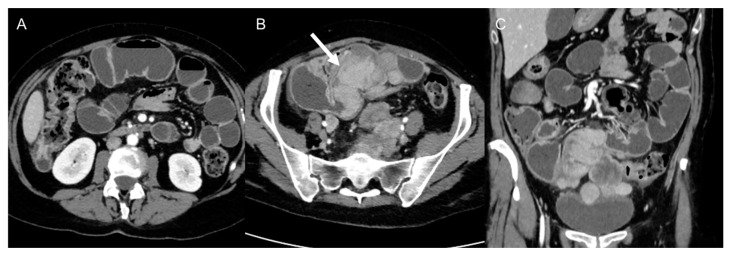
Bowel obstruction in 38-year-old woman with metastatic leiomyosarcoma. Axial (A,B) and coronal (C) CT images on the venous phase show dilatation of small bowel loops with air–fluid levels secondary to the presence of metastatic masses (arrow) in the pelvis.
